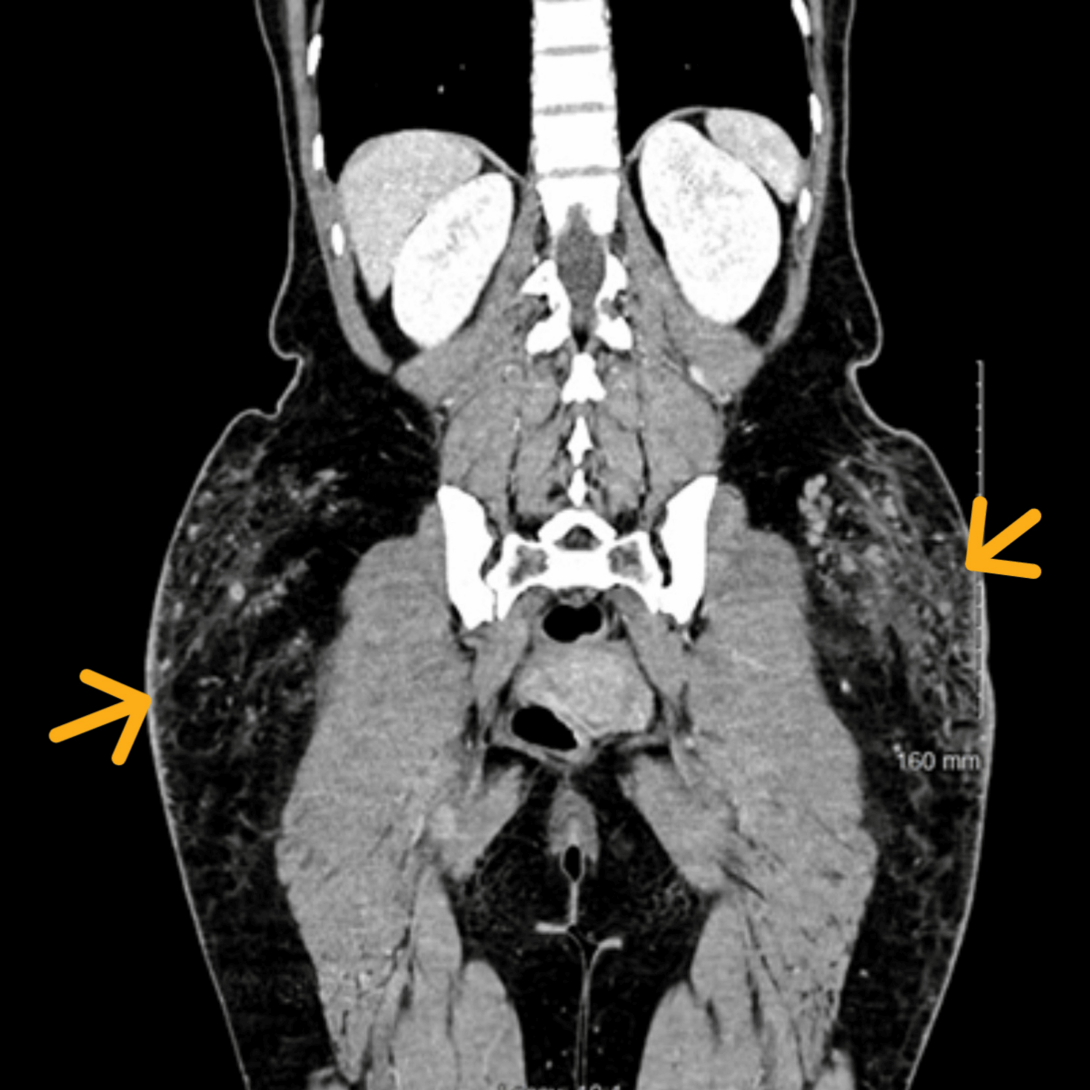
Coronal CT with contrast. Yellow arrows pointing at the extensive area of free material within the overlying connective tissue induration.
